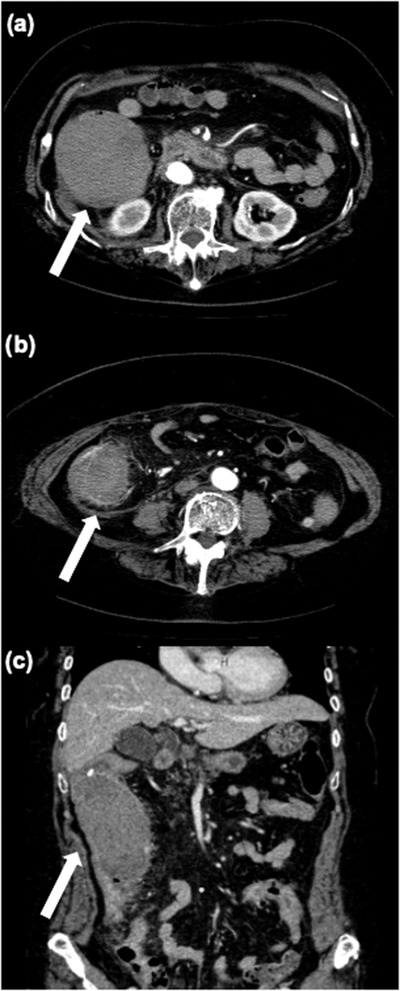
Contrast‐enhanced computed tomography performed immediately after urgent endoscopy the day after cold snare polypectomy: (a) a 70‐mm hematoma (white arrow) observed at the ascending colon; (b) a dirty fat sign visible around the ascending colon (white arrow); and (c) coronal view of dirty fat sign around the ascending colon (white arrow)
